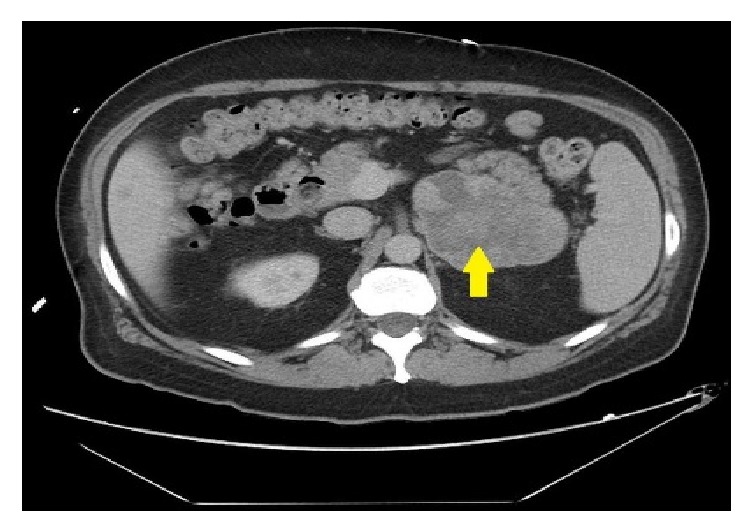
CT of abdomen showing 8.1 cm heterogeneous left adrenal mass.
